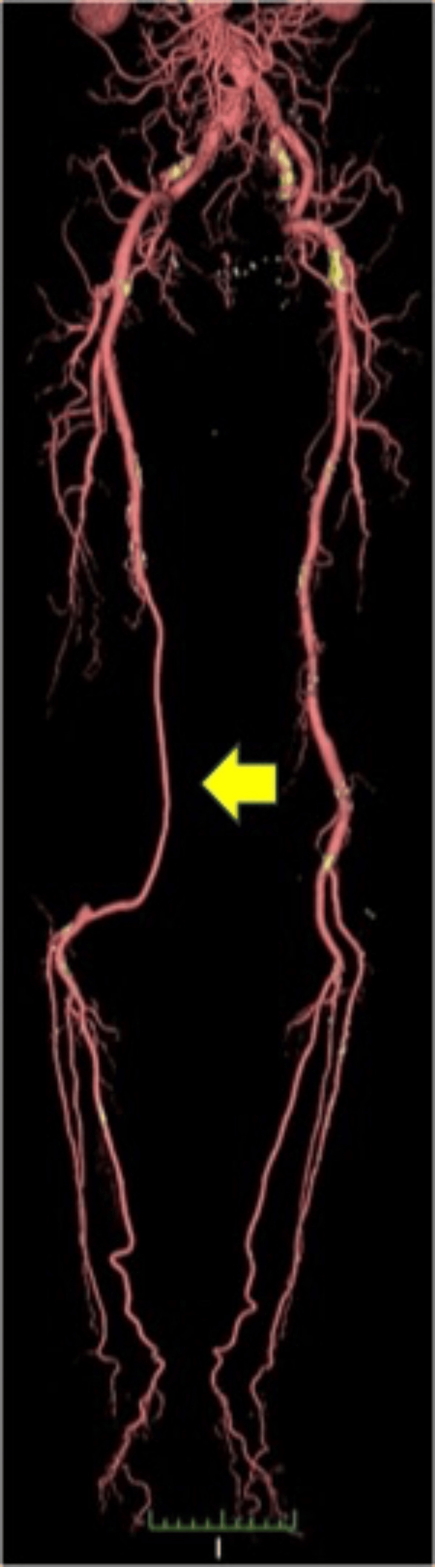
Postoperative three-year 3D computed tomography (CT) scan of arteries of the lower extremities This image shows that the bypass of the great saphenous vein flows well with no evidence of a recurrent aneurysm (arrow).
